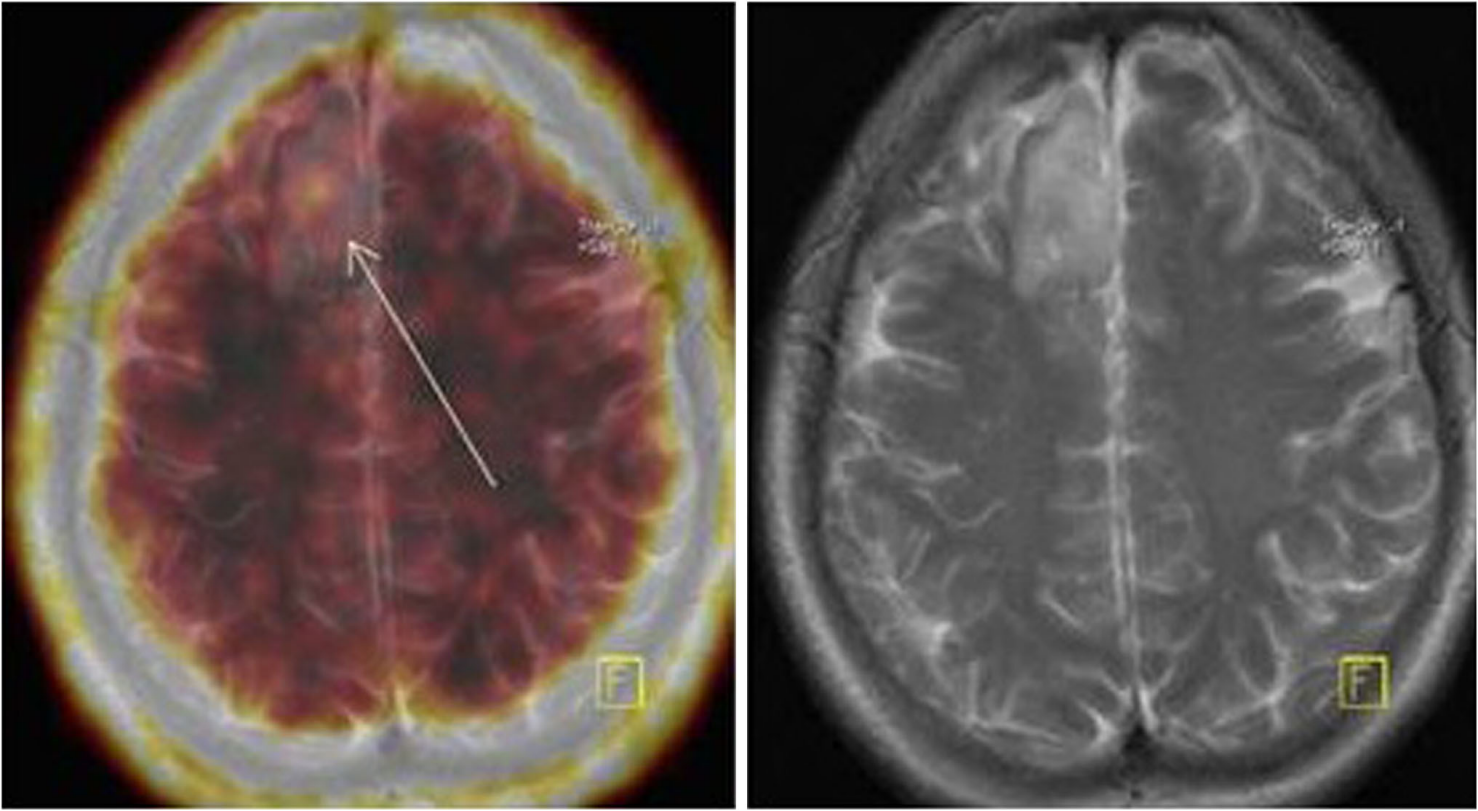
Grade II oligodendroglioma recurrence (*arrow*), low ^18^F-FLT uptake (SUV_max_ 0.85, tumor-to-background ratio 7.0). FLT* fluorothymidine, *SUV* standardized uptake value
